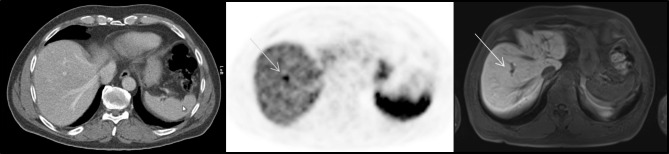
False-positive PET finding in a 65-year-old man with a NET of the caecum. In the contrast-enhanced CT (left) no suspicious findings of the liver were detected; on PET (middle) focal SSR-uptake above SSR-uptake of liver parenchyma was detected on hepatobiliary phase MRI (right), no suspicious liver lesions were found, most likely due to bile duct-associated SSR-uptake.
